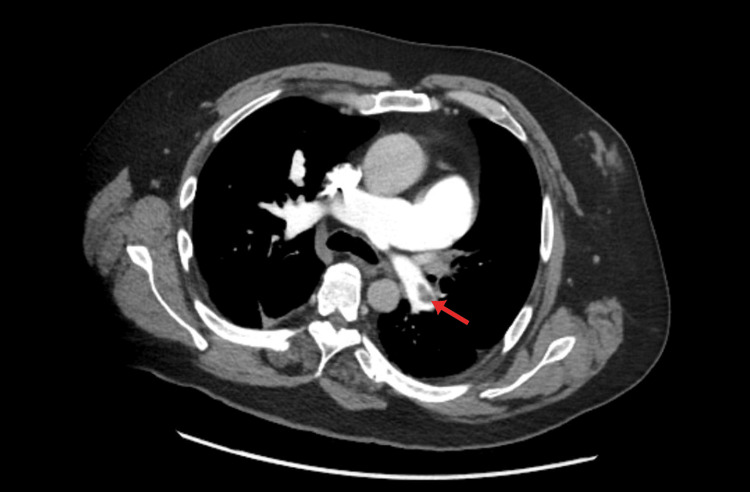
Axial computed tomography angiography showing a segmental left pulmonary embolus. Repeat computed tomography angiography of the chest, abdomen, pelvis, and run-off confirming the presence of a pulmonary embolus (red arrow), suggesting an underlying concurrent venous thromboembolism process.
